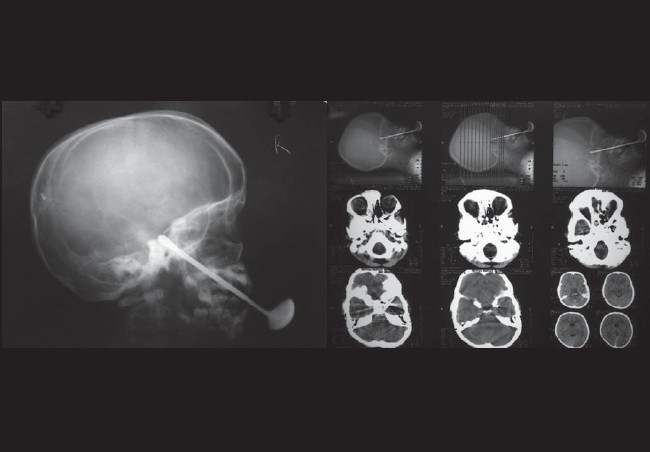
X-ray skull and CT scan of brain and orbit showing entry of spoon through hard palate with intracranial extension
